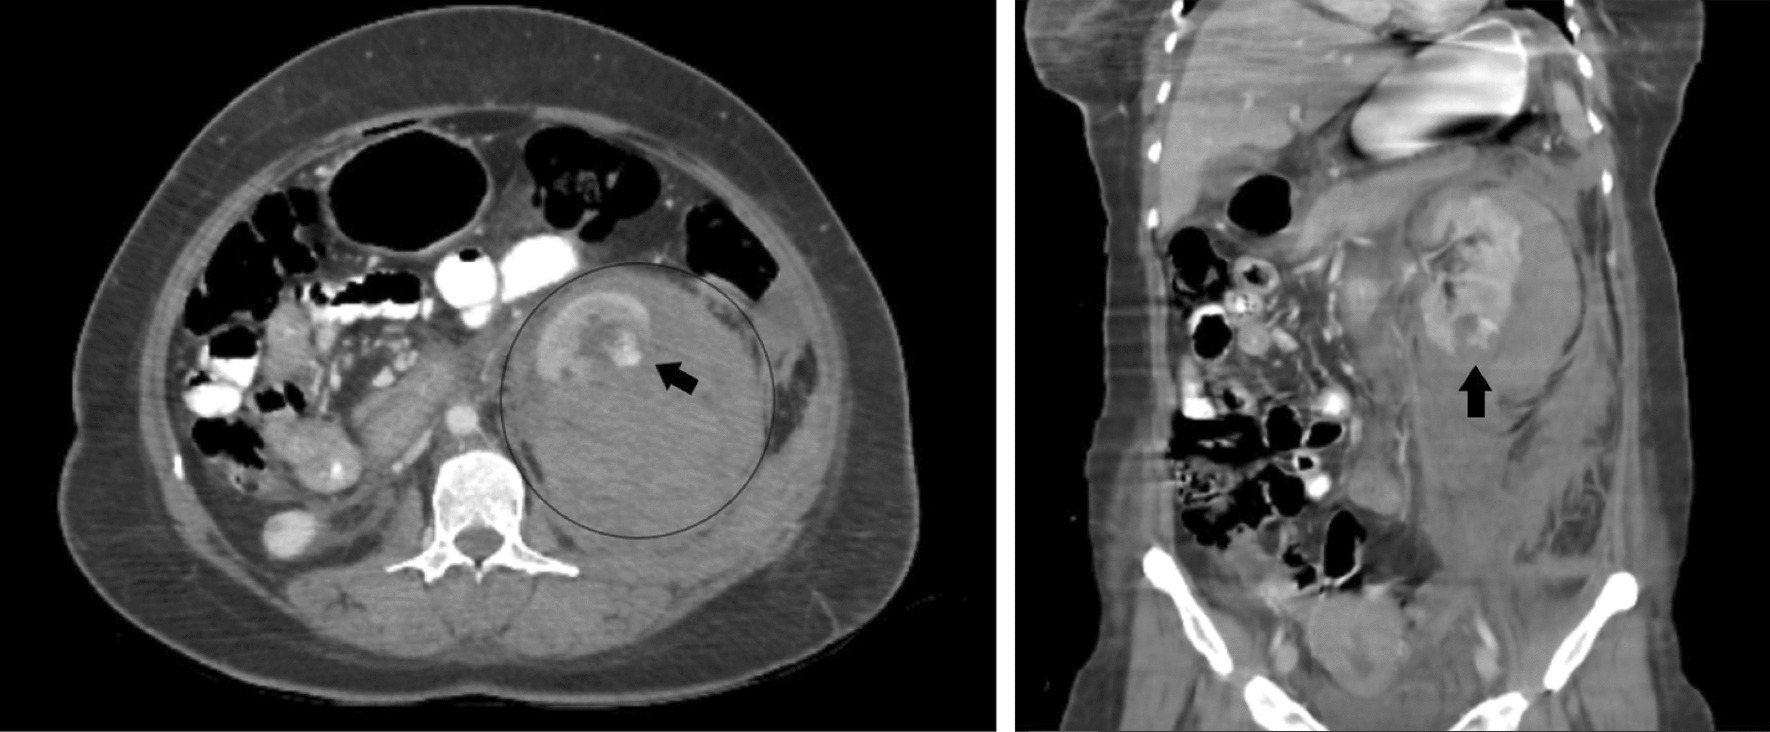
Abdominopelvic computed tomography scan with intravenous contrast in the axial (left) and coronal (right) planes. A hypodense lesion (black arrow) with foci of intense enhancement and active bleeding was identified in the lower pole of the left kidney. Additionally, the lesion was surrounded by a hematoma (black circle) with a pressure effect, leading to an anterior displacement of the left kidney
